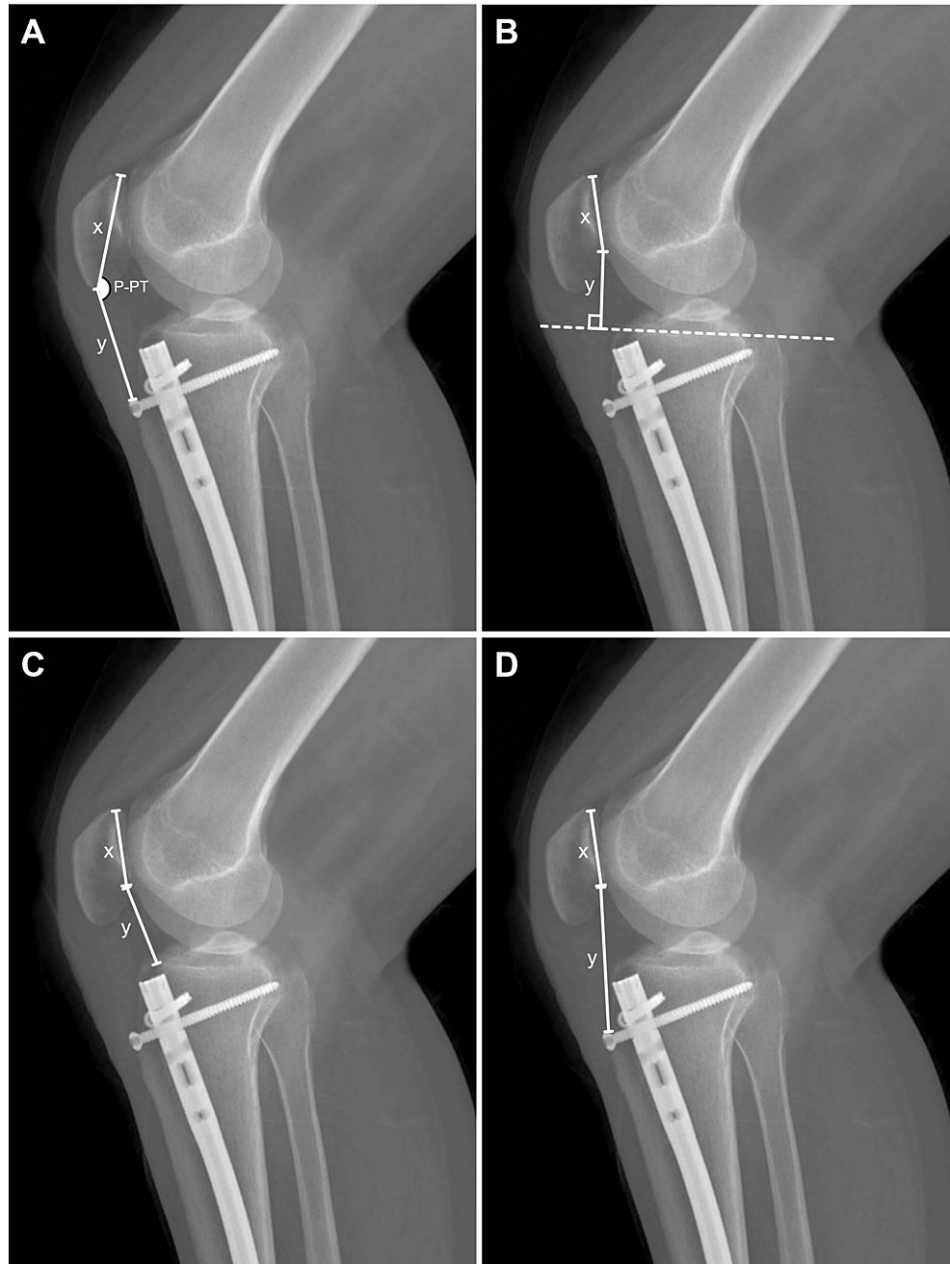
Sample measurements of patellar height indices and sagittal angulation on the lateral direct radiograph of a patient who underwent reamed intramedullary nailing for a tibial shaft fracture: (A) Insall-Salvati index and patella-patellar tendon angle, (B) Blackburne-Peel index, (C) Caton-Deschamps index, and (D) modified Insall-Salvati index.
